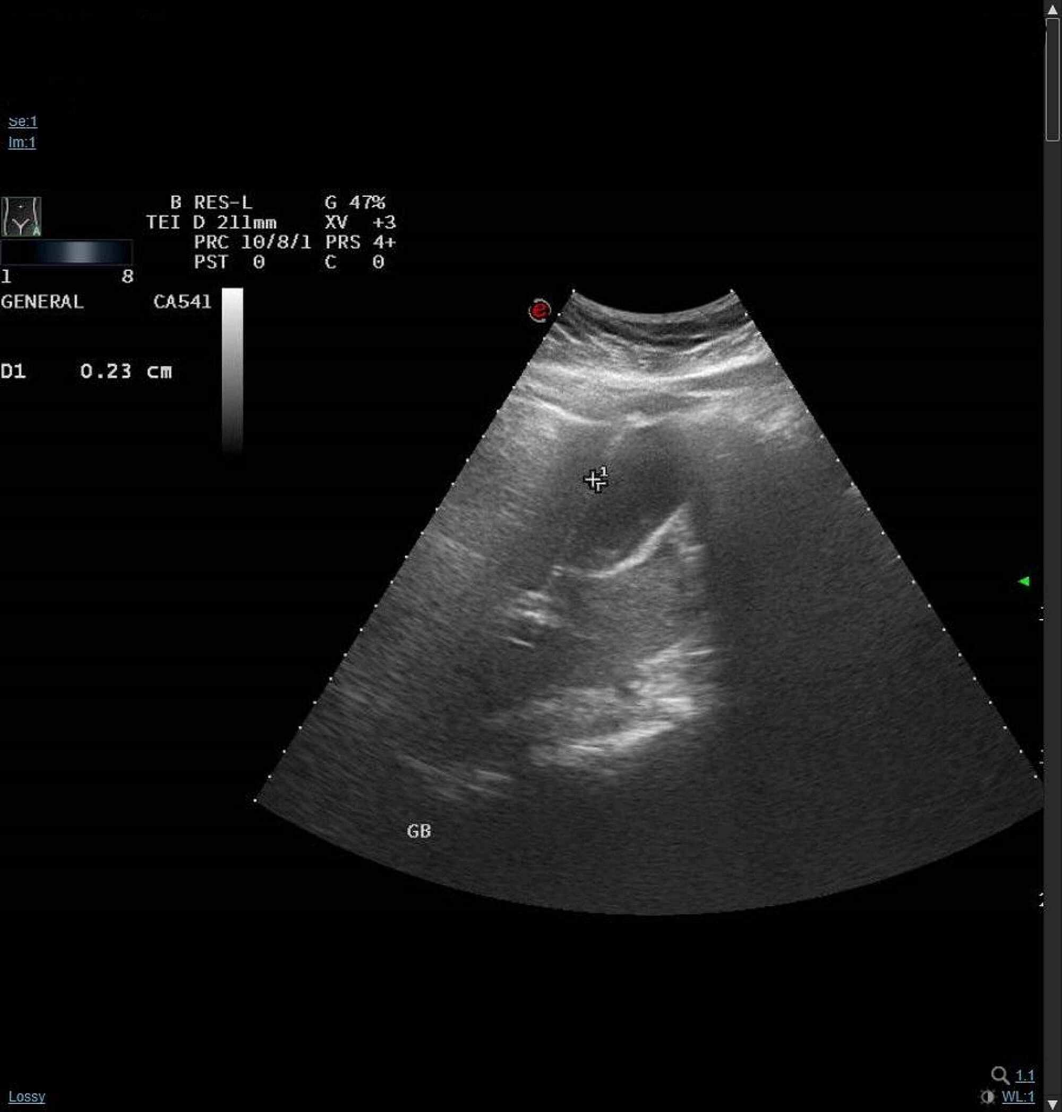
Ultrasound of gallbladder showing no significant changes.
